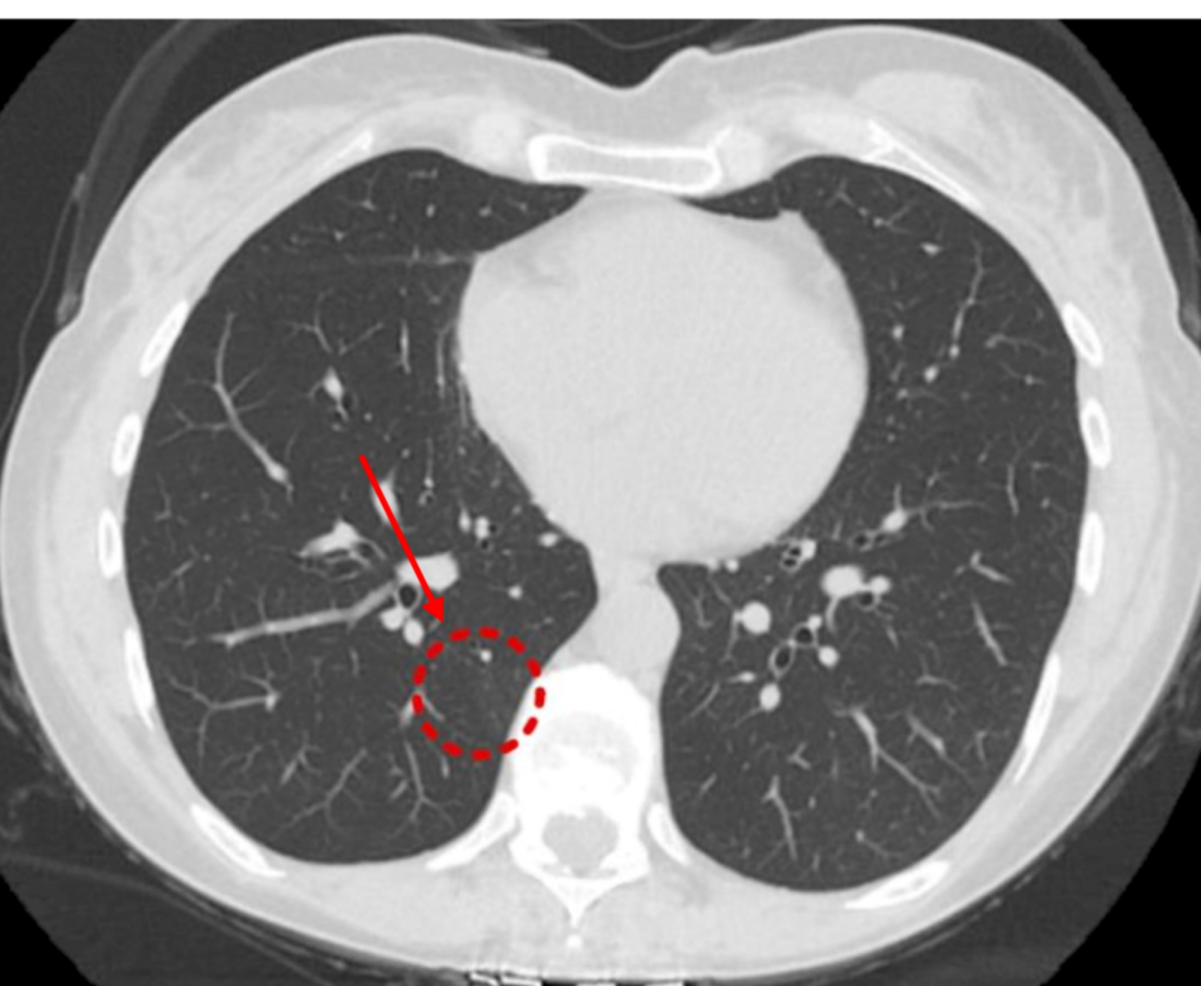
Non-contrast computerized tomography (NCCT) of the chest (three months from previous CT) Interval resolution of ground-glass opacity in the right lower lobe
